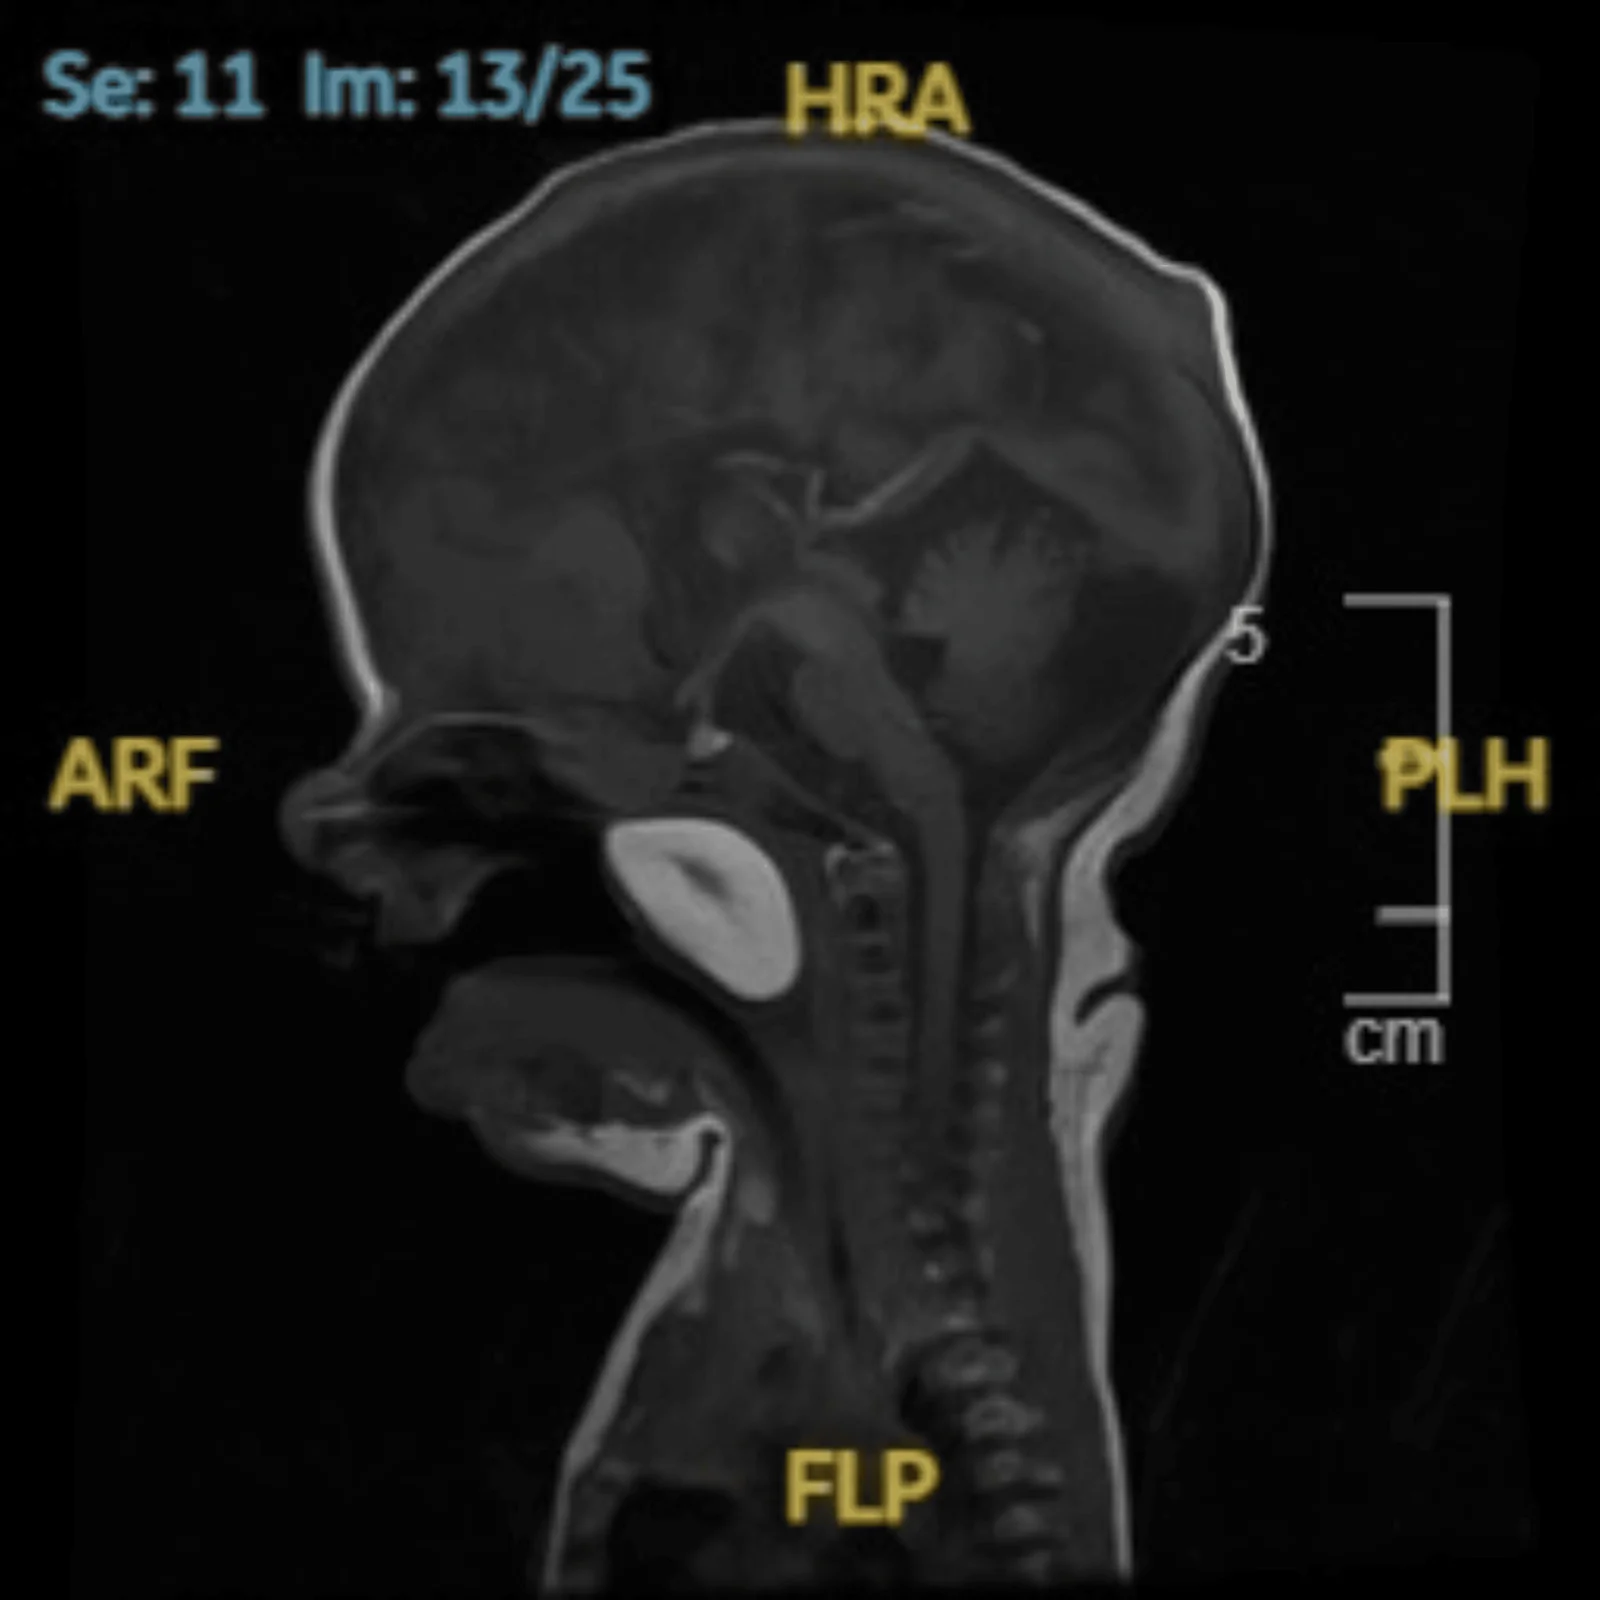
T1-weighted MRI scan showing hairy polyp (sagittal view) MRI: magnetic resonance imaging
